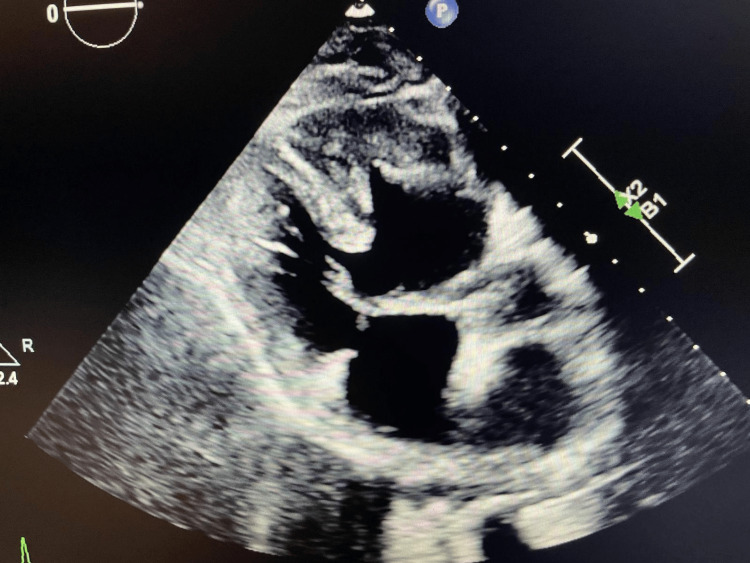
TTE showing hypoplastic left heart post-Fontan procedure and dextrocardia TTE, transthoracic echocardiogram
